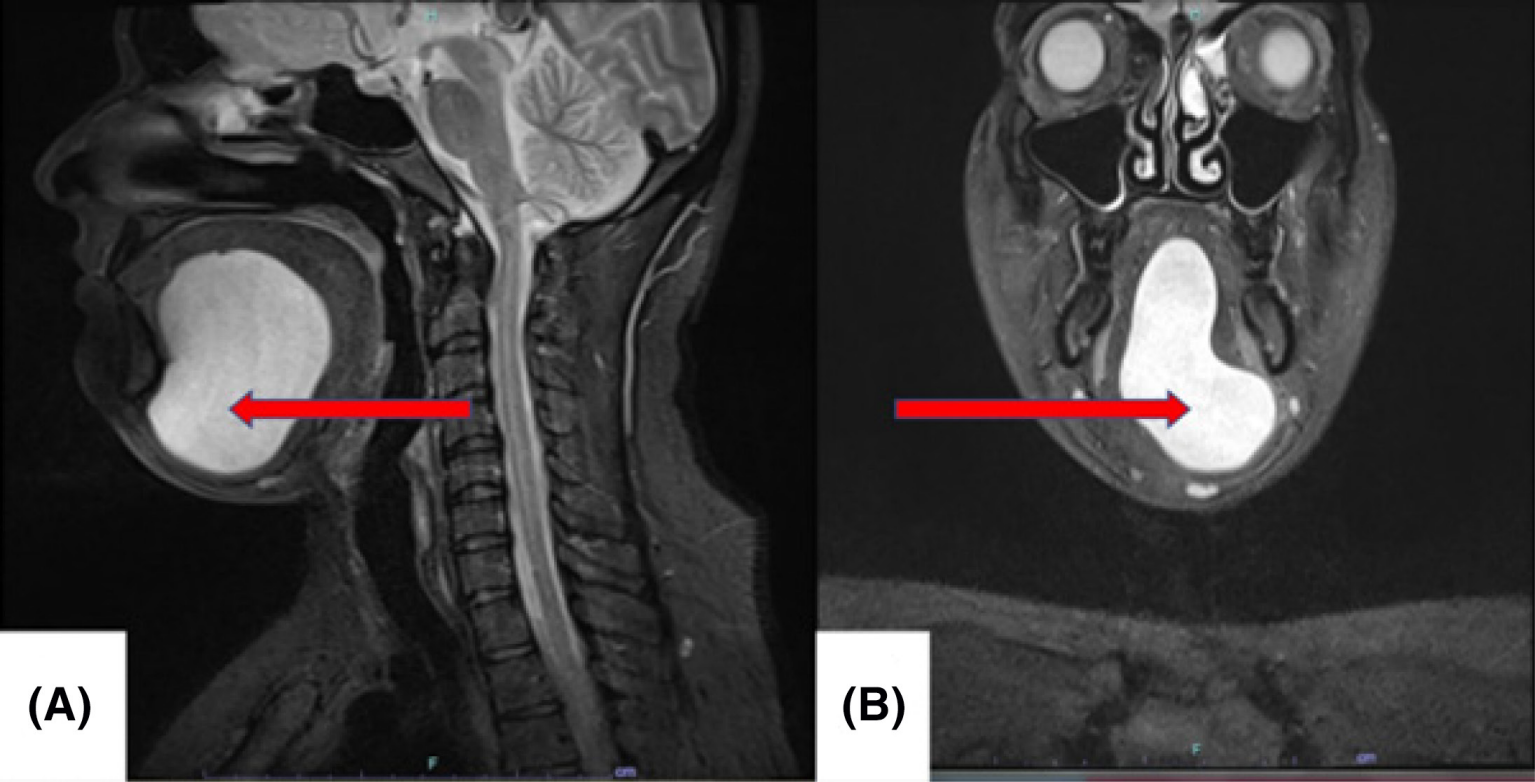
(A and B) T‐2 weighted MRI sagittal section and axial view showing the extent of the swelling.
